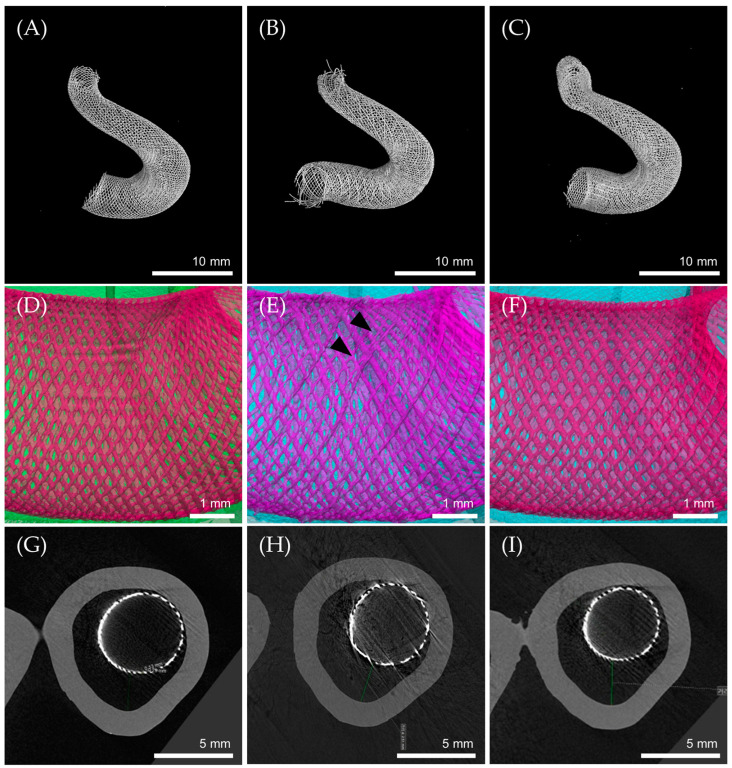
Micro CT image (A–C): flow diverter CT image, (D–F): wire arrangement, (G–I): aneurysm cross-section, (A,D,G): NiTi FD, (B,E,H): NiTi-Pt/W FD, (C,F,I): NiTi(Pt) FD.
